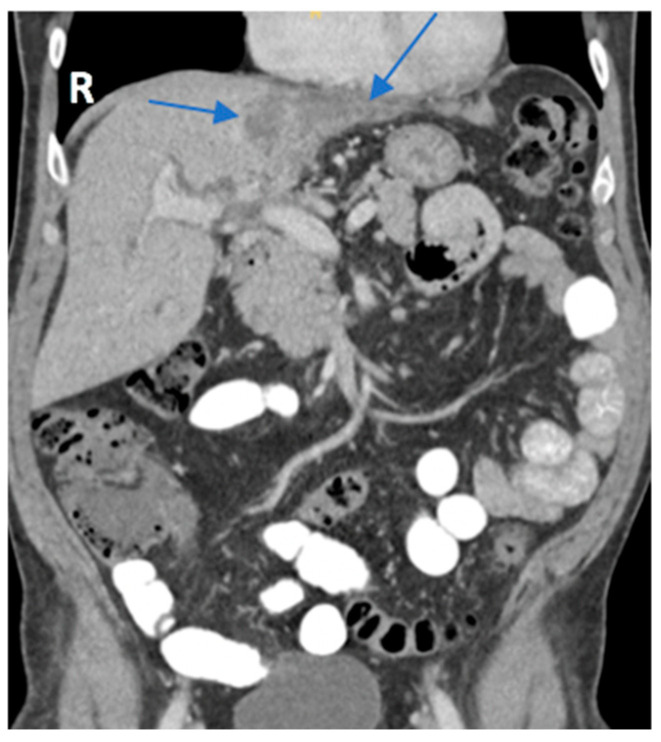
Coronal view of the CT of the abdomen and pelvis with contrast showed irregular enhancement in the right upper quadrant, suggestive of an intrahepatic cholangiocarcinoma with involvement of the left hepatic lobe (blue arrows). The primary tumor metastasized to the umbilicus and presented as an umbilical mass found on examination of the patient.
